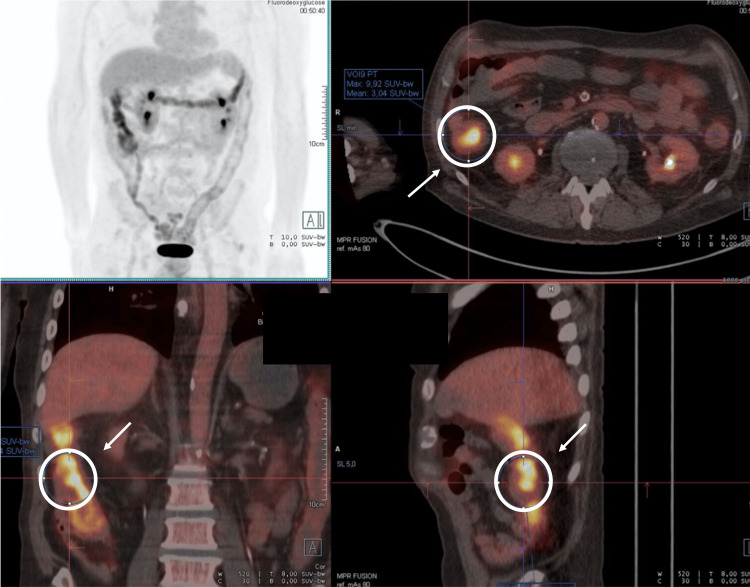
PET scan showing increased FDG uptake in the ascending colon, with no other findings explaining the elevated inflammatory markers
